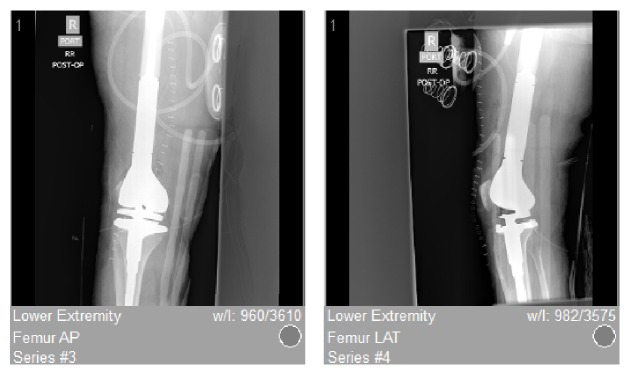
Figure 3 shows postoperative AP/lateral radiographs status, after wide resection of the lesion and replacement with distal femoral endoprosthesis.
